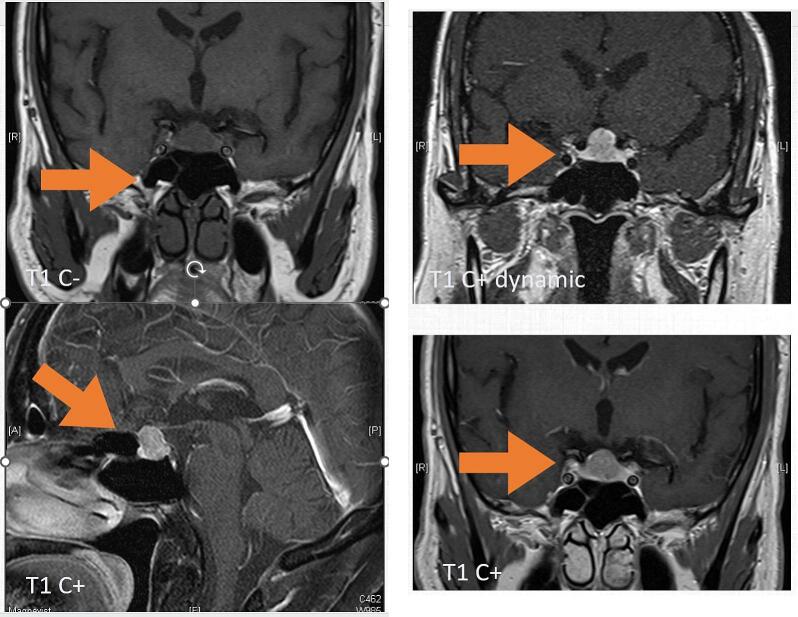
Pre-operative MRI of Case 1.
